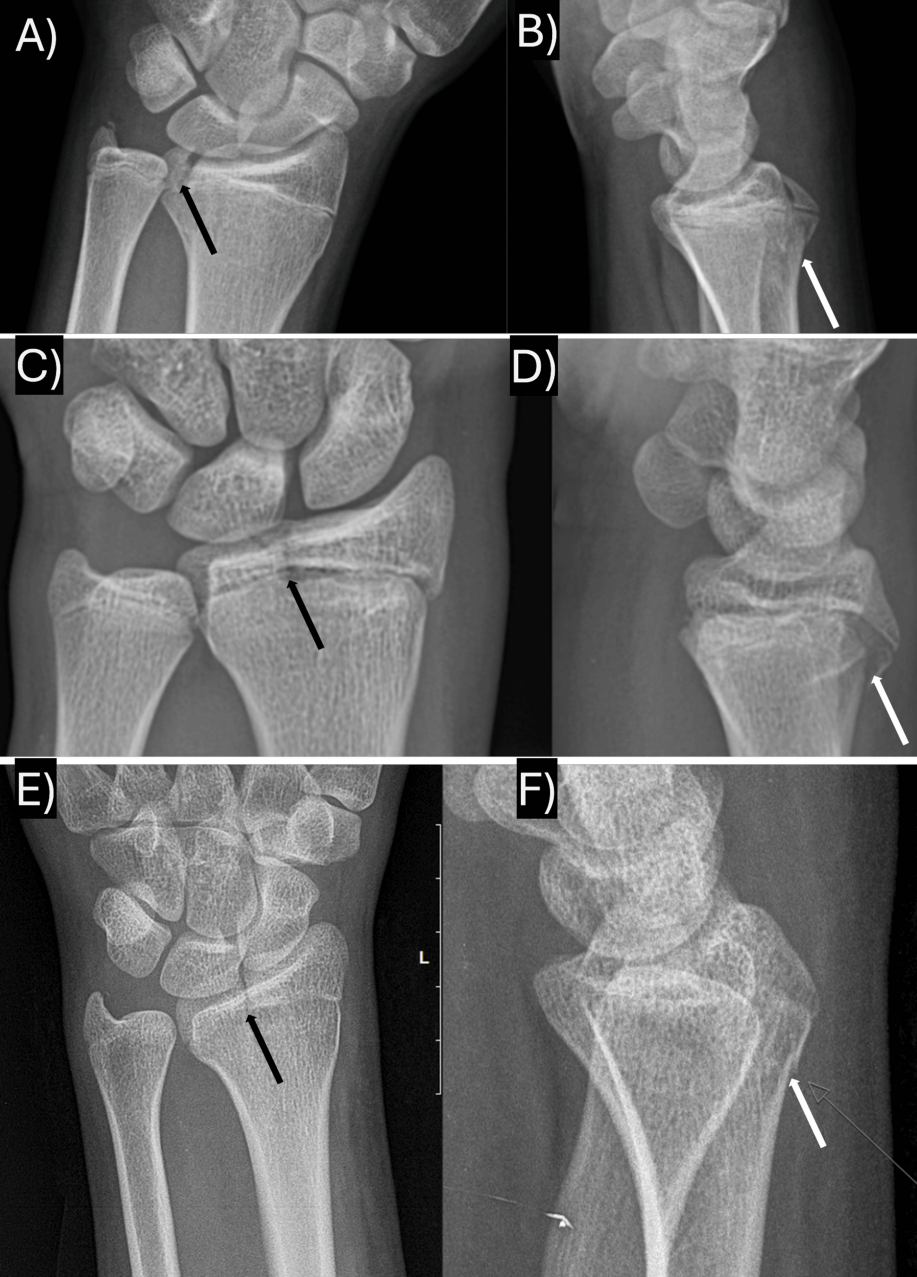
Injury films of cases with SH-III components on AP radiographs and SH-II components on lateral radiographs A) Case I's AP radiographs; B) Case I's lateral radiographs; C) Case IV's AP radiographs; D) Case IV's lateral radiographs; E) Case VI's AP radiographs; F) Case VI's lateral radiographs. In all panels, the black arrows demonstrate the AP radiograph SH-III component and the white arrows demonstrate the lateral radiograph SH-II component. SH: Salter-Harris; AP: anterior-posterior
